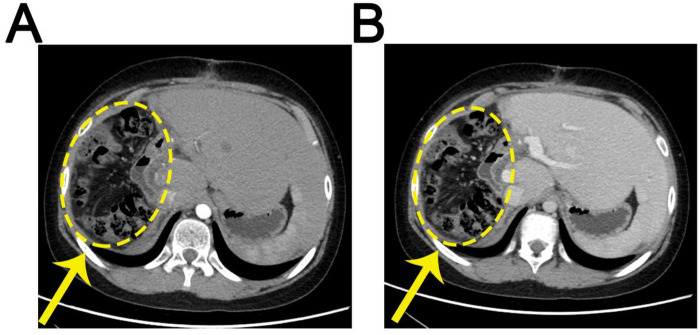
Post-operative CT scans evaluating the surgical outcome and hepatic status. (A) Arterial phase. (B) Venous phase. Both images show the resection site and surrounding hepatic structures, with the previous tumor location roughly indicated. The yellow dotted circles roughly indicate the previous tumor location.
